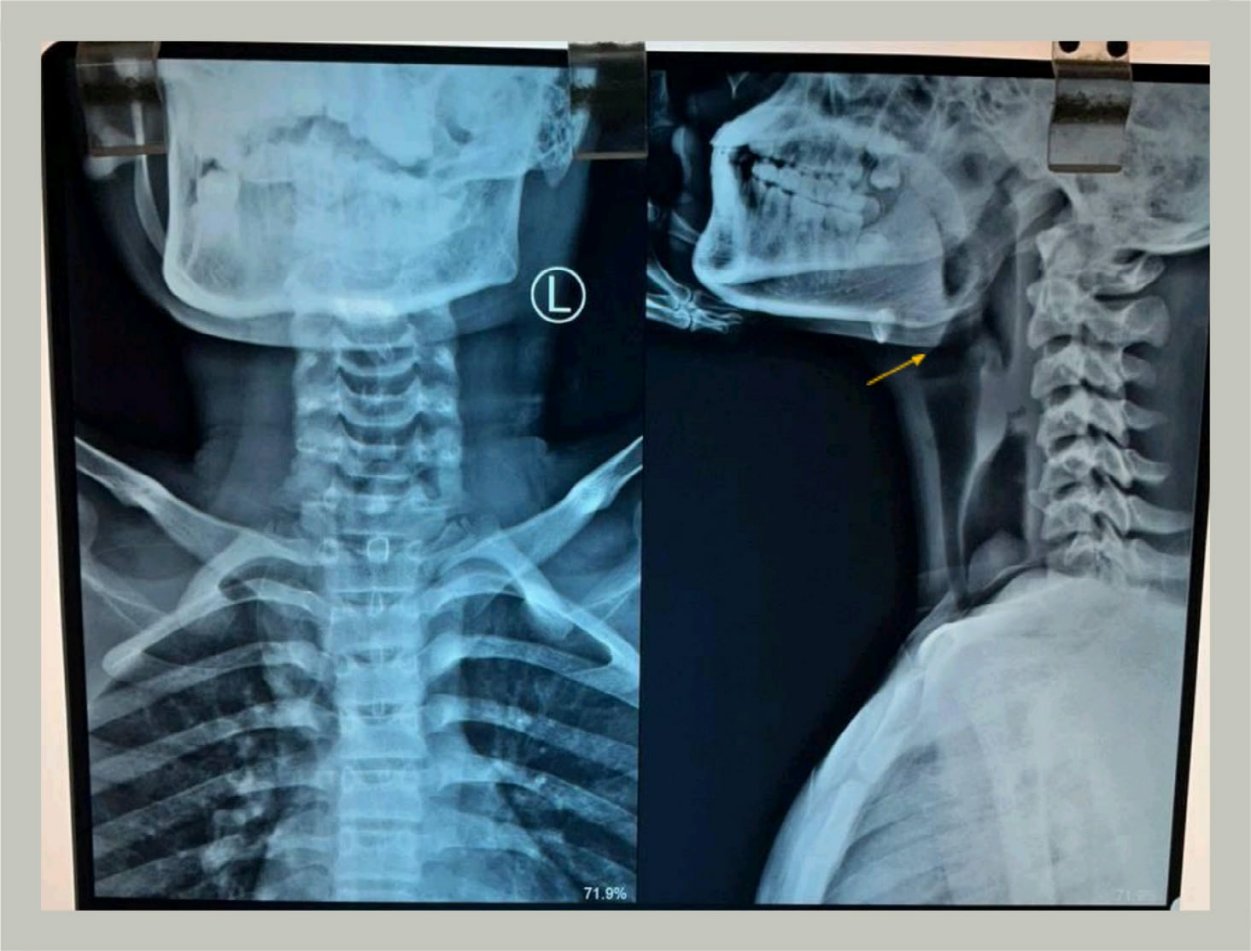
Anterioposterior (left) and lateral (right) radiographic views of head, neck, and upper chest.
